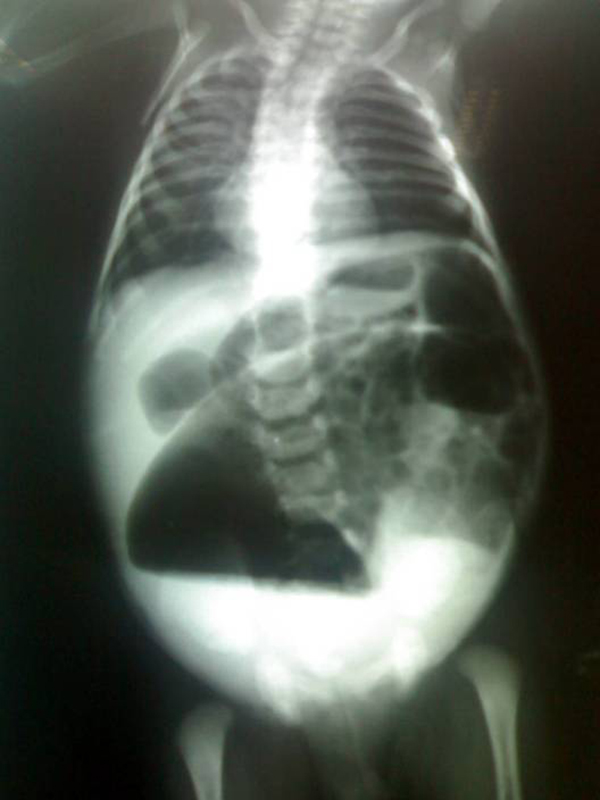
An erect abdominal radiograph showing a dilated bowel loop with an air fluid level occupying more than half of the diameter of the abdomen.
